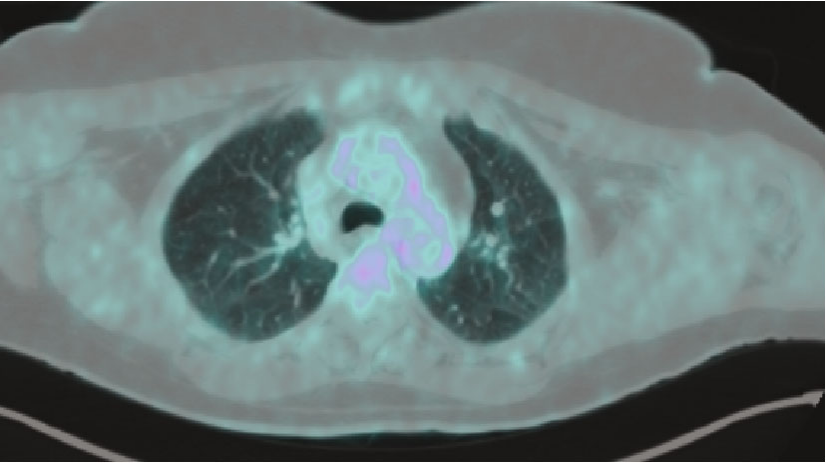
PET-CT body scan response after pembrolizumab suspension.
